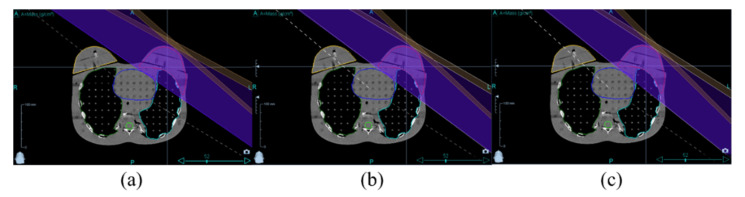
Example of TD plans in two directions with RAO {(a) 308° (b) 310° (c) 312°)} and LPO {(a) 121° (b) 123° (c) 125°)} in axial CT images with ROIs (PTV and OARs) for left-breast cancer.
